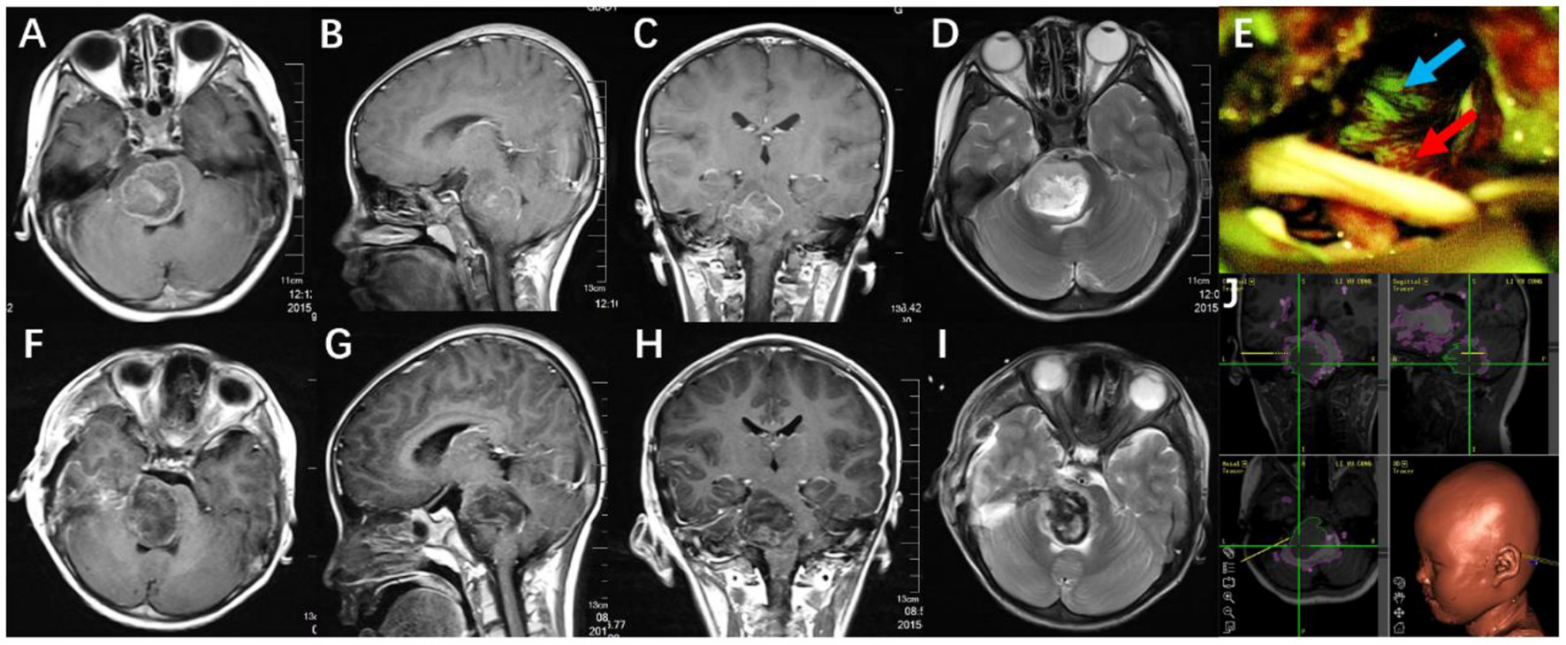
(A–D) Preoperative MRI scan (axial, coronal, sagittal T1-weighted with gadolinium, and T2-weighted) showed a pediatric anaplastic astrocytoma in the right pons. (F–I) Postoperative MRI showed subtotal tumor resection. (E) Tumor was visualized under the YELLOW 560 nm filter (blue arrow showed tumor, red arrow showed brain tissue). (J) The surgical plan was made with the assistance of neuronavigation, revealing the relationship between the tumor, white matter tracts, and brainstem nuclei.
